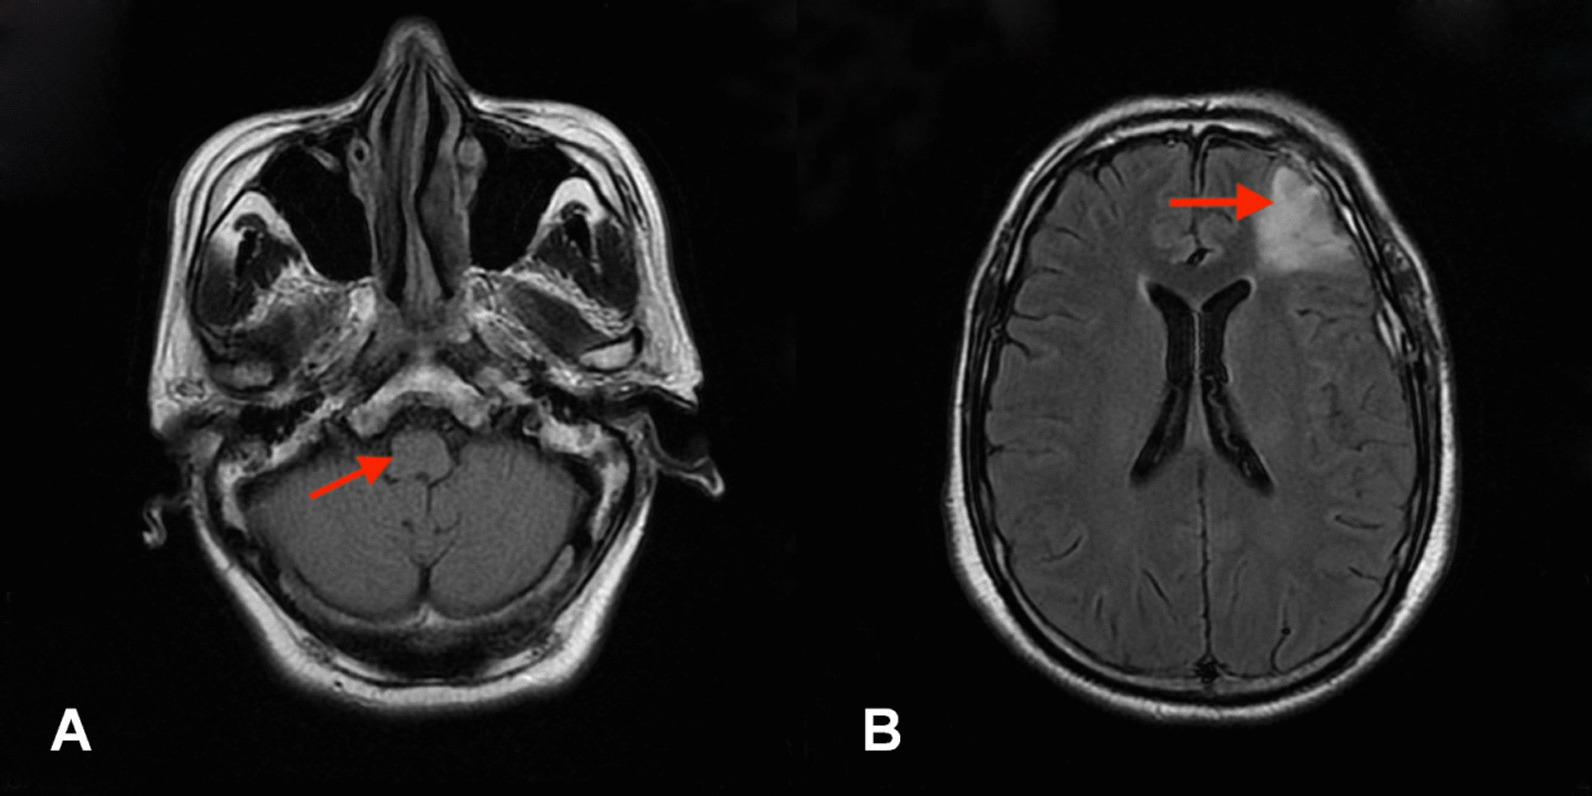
Magnetic resonance imaging. A No lesion concerning the brainstem (arrow, left). B Symptoms cannot be explained by left frontal lobe malacia and subdural hematoma (arrow, right)
